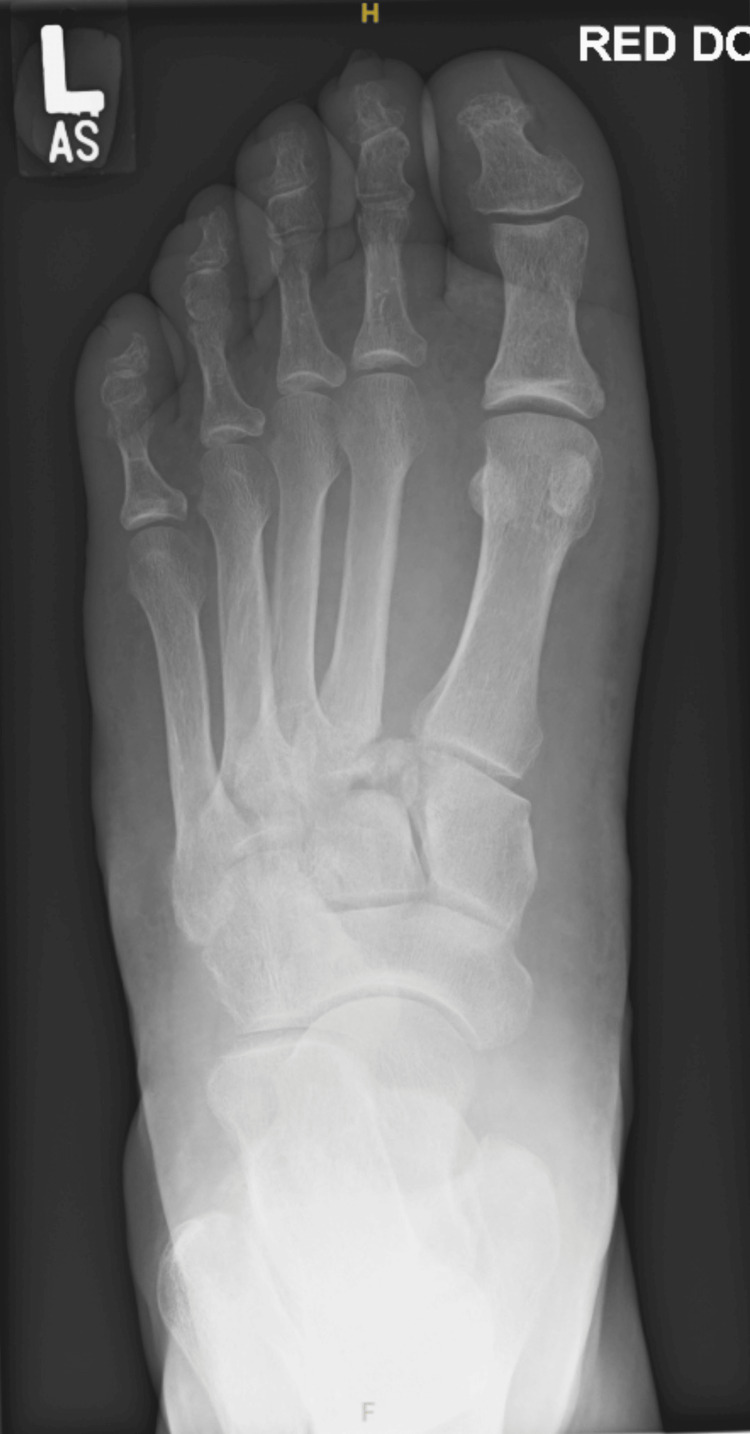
Plain radiograph of the left foot demonstrating widening of the first-second intermetatarsal space with a displaced fracture at the base of the second metatarsal, consistent with a Lisfranc injury.
